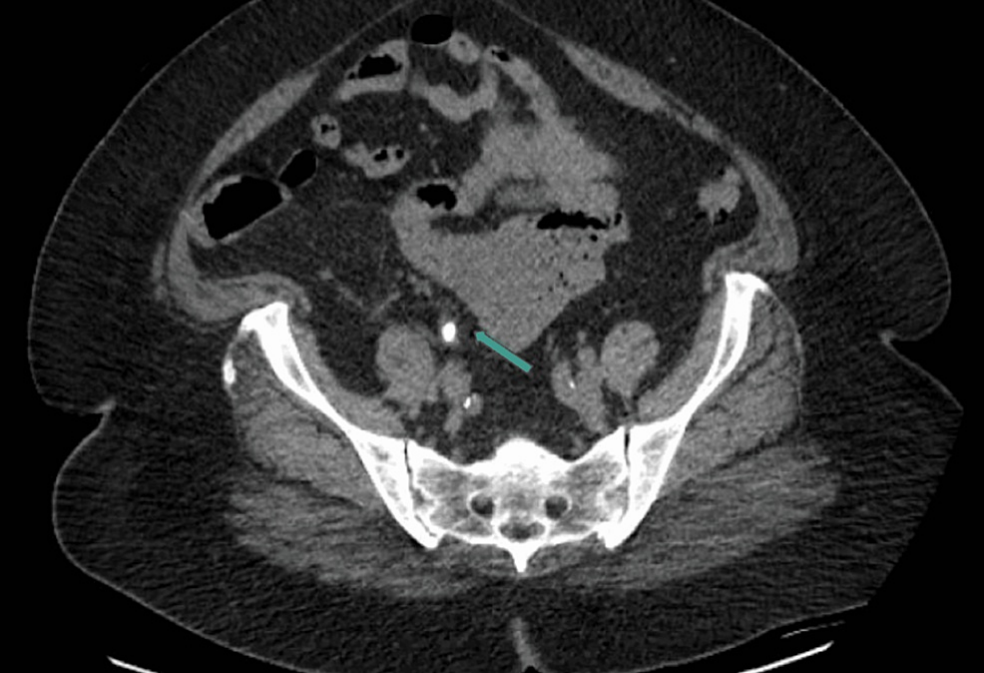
CT transverse view demonstrating obstructive ureteral calculi CT: computed tomography
